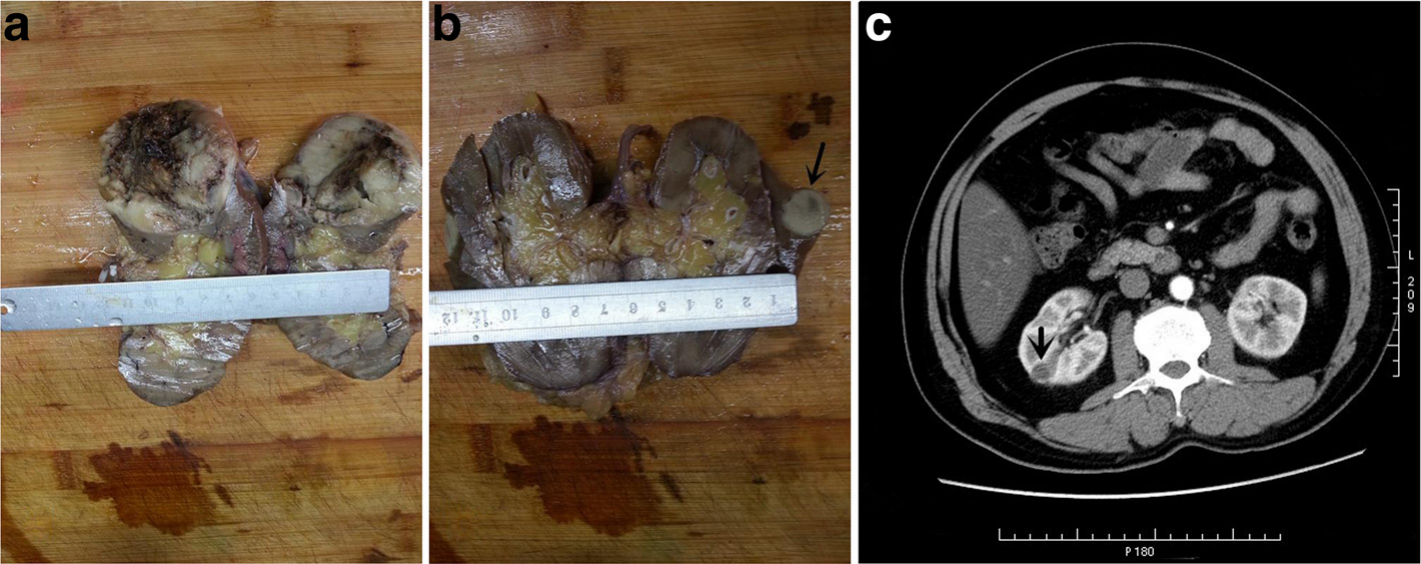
Macroscopic observation and CT findings of MTSC and sPRCC. (a): MTSC (case 2) showed a well-defined nodular mass with a greyish-white cut surface accompanied with hemorrhage and necrosis. (b): sPRCC (case 3) showed a well-defined nodular mass with a greyish-white cut surface (arrow). c): Computed tomography revealed a mass in the renal cortex in case 3 that was well-defined (arrow).
